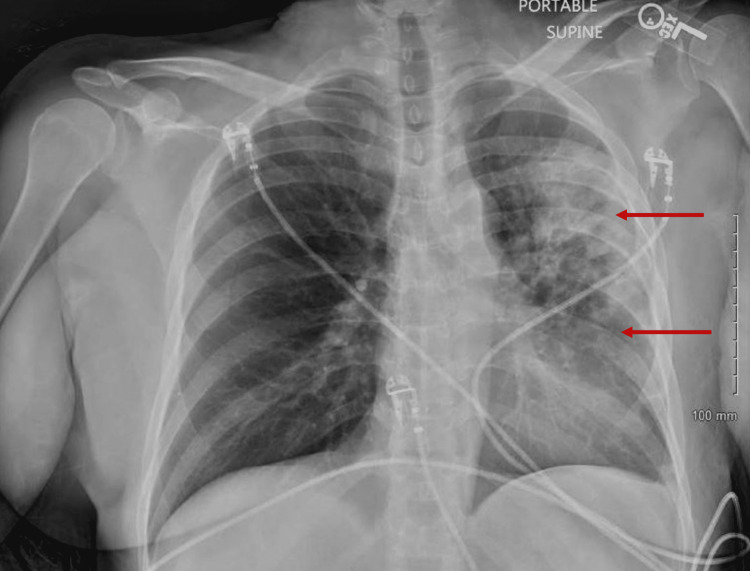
Chest X-ray As noted by the red arrows, the chest x-ray was significant for extensive diffuse left hemithorax consolidation, consistent with pneumonia.
